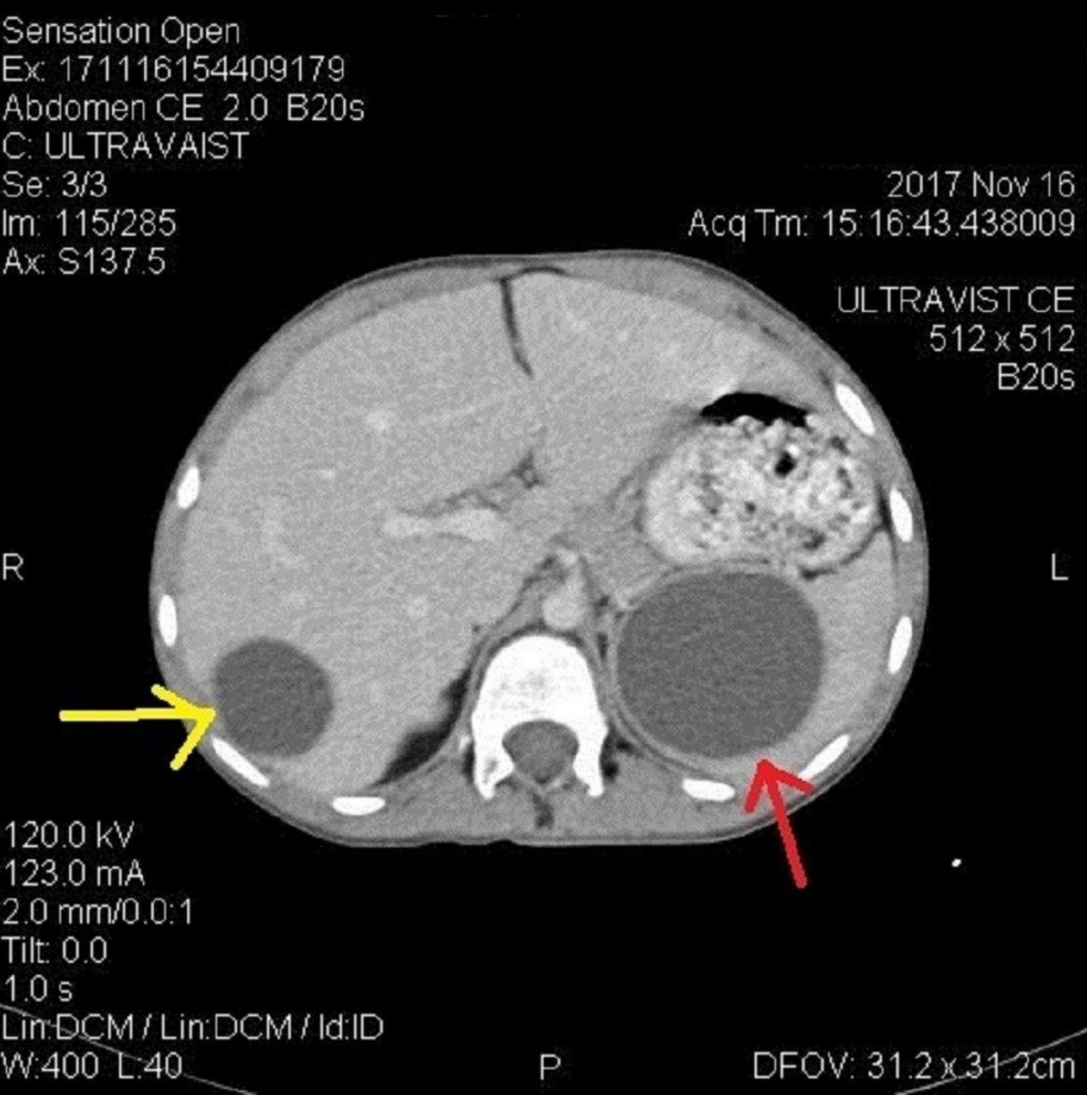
Computed tomography (CT) findings CT abdomen showing cyst in liver (yellow) and cyst in spleen (red).
